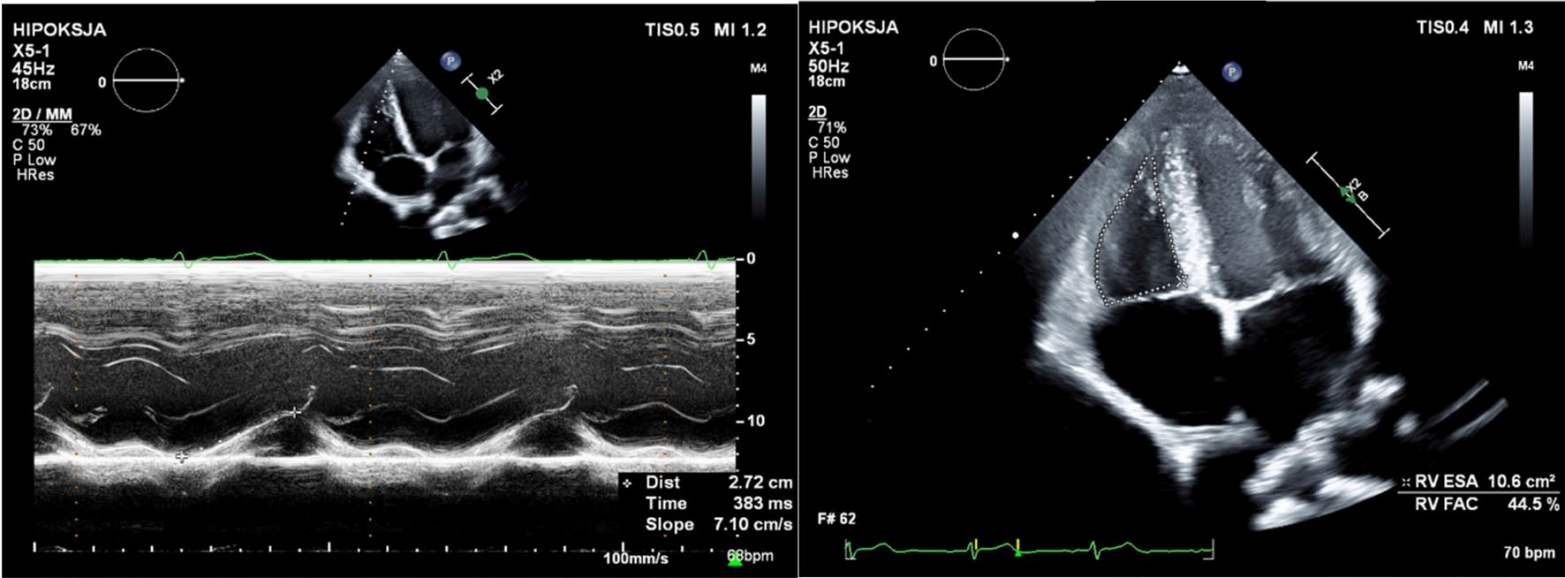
Examples of echocardiographic assessment of the RV function in an untrained participant at rest. Left panel—measurement of tricuspid annular plane systolic excursion (TAPSE) using the M‐mode method (TAPSE: 27 mm). Right panel—tracing of the RV endocardial border in the apical 4‐chamber view in end‐systole, with a calculated fractional area change (FAC) of 44.5%.
